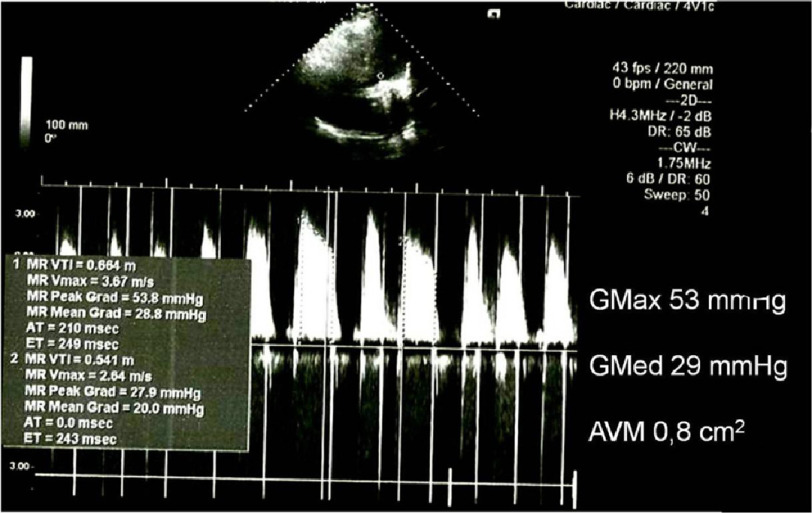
Doppler study of the mitral valve prosthesis revealed a maximum and mean transvalvular gradient of 53 and 29 mmHg, respectively.
